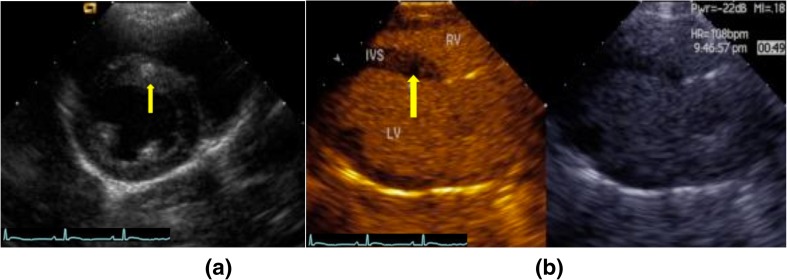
a A two-dimensional image showing the position of ablation (*arrows*) on the ventricular septum in LV short-axis view. Hyper-echo region indicates the ablated region. b Contrast mode in parasternal LV long-axis view, *LV* left ventricle, *RV* right ventricle, *IVS* interventional septum. Defect in blood filling is found at the ablation site in the *left panel* of Fig. 2b
